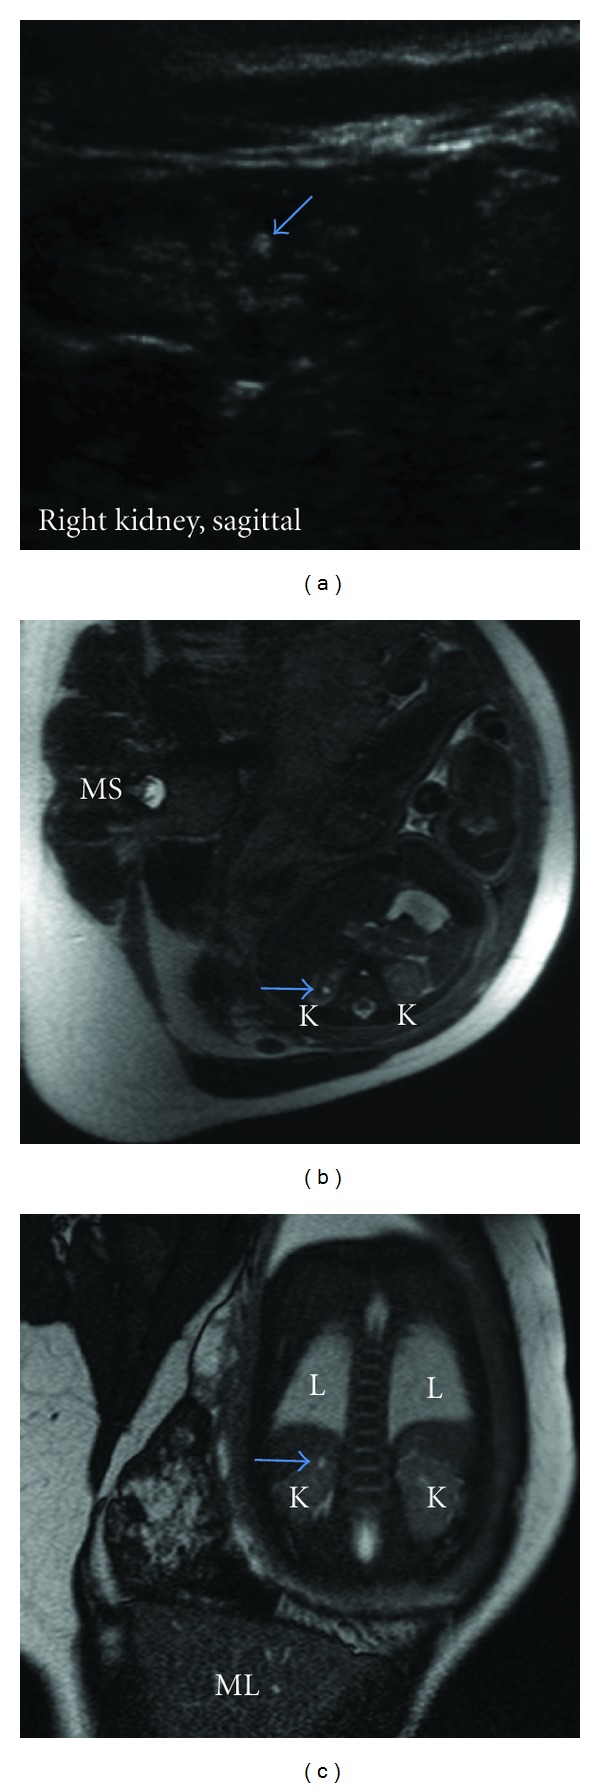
(a) On ultrasound, a single small, echogenic lesion was detected within the parenchyma of the upper pole of the right kidney, consistent with an angiomyolipoma. (b) T-2-weighted MRI image, in the transverse plane, at the level of the top of the right kidney demonstrates a high-signal lesion within the upper pole (arrow). K, kidneys; MS, maternal spine. (c) TruFISP coronal image through the kidneys shows another view of the same lesion (arrow) K, kidney; ML, maternal liver; L, lung.
